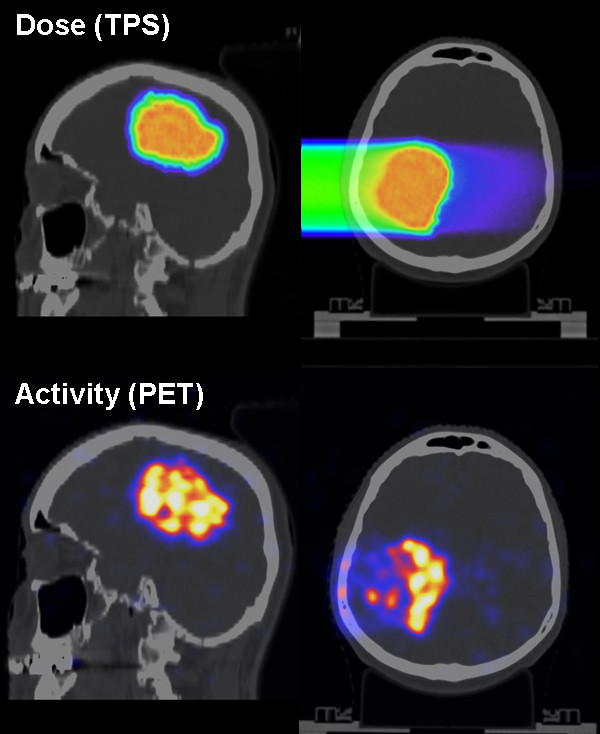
Patient undergoing a PET/CT measurement after scanned ion irradiation at HIT. The patient was treated for a primary brain tumour with a carbon ion boost. The top panel depicts the dose planned by the commercial TPS overlaid onto the planning CT in axial and sagittal views. The bottom panel depicts the PET image acquired ca. 8 minutes after irradiation and overlaid onto the control CT data, merged and visualised approximately in the same views as the top raw.
